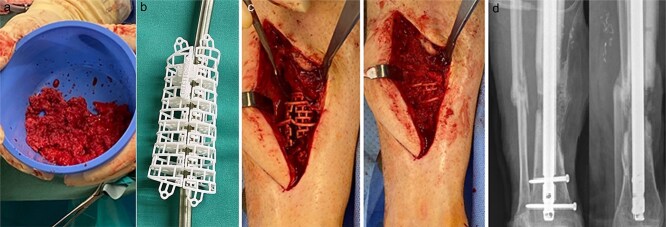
(a) RIA-harvested bone graft; (b) graft cage; (c) operation site with graft cage; (d) postoperative X-ray after graft cage implantation.
